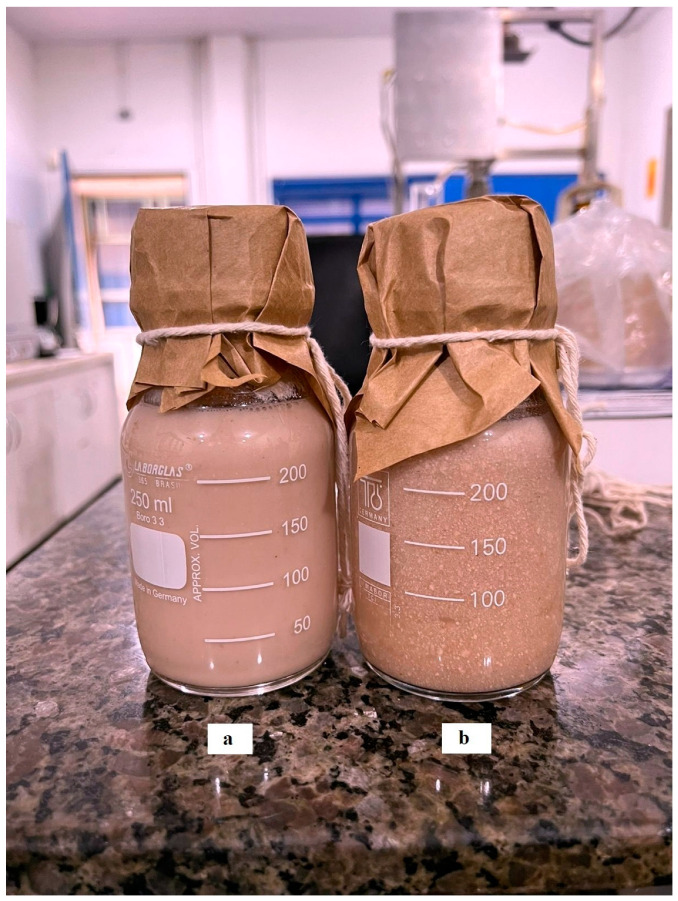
(a) Ultrasound-treated (U7.5) and (b) non-treated probiotic beverages.
